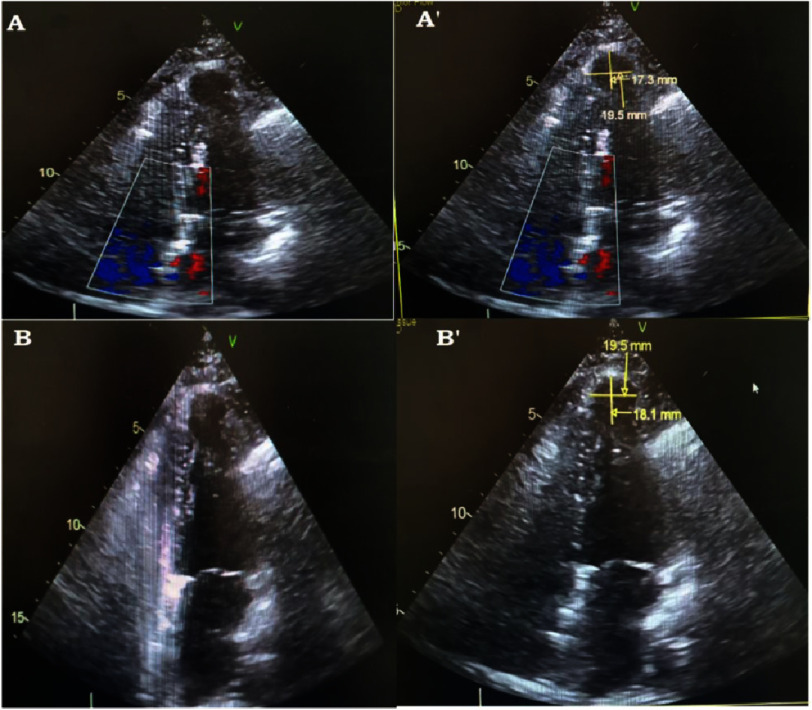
Contrasted echocardiography showing apical aneurysm aspect of the left ventricle measuring 17.3 mm × 19.5 mm in apical four chamber (A’) and 19.5 mm × 18.1 mm in apical two chamber (B’).
